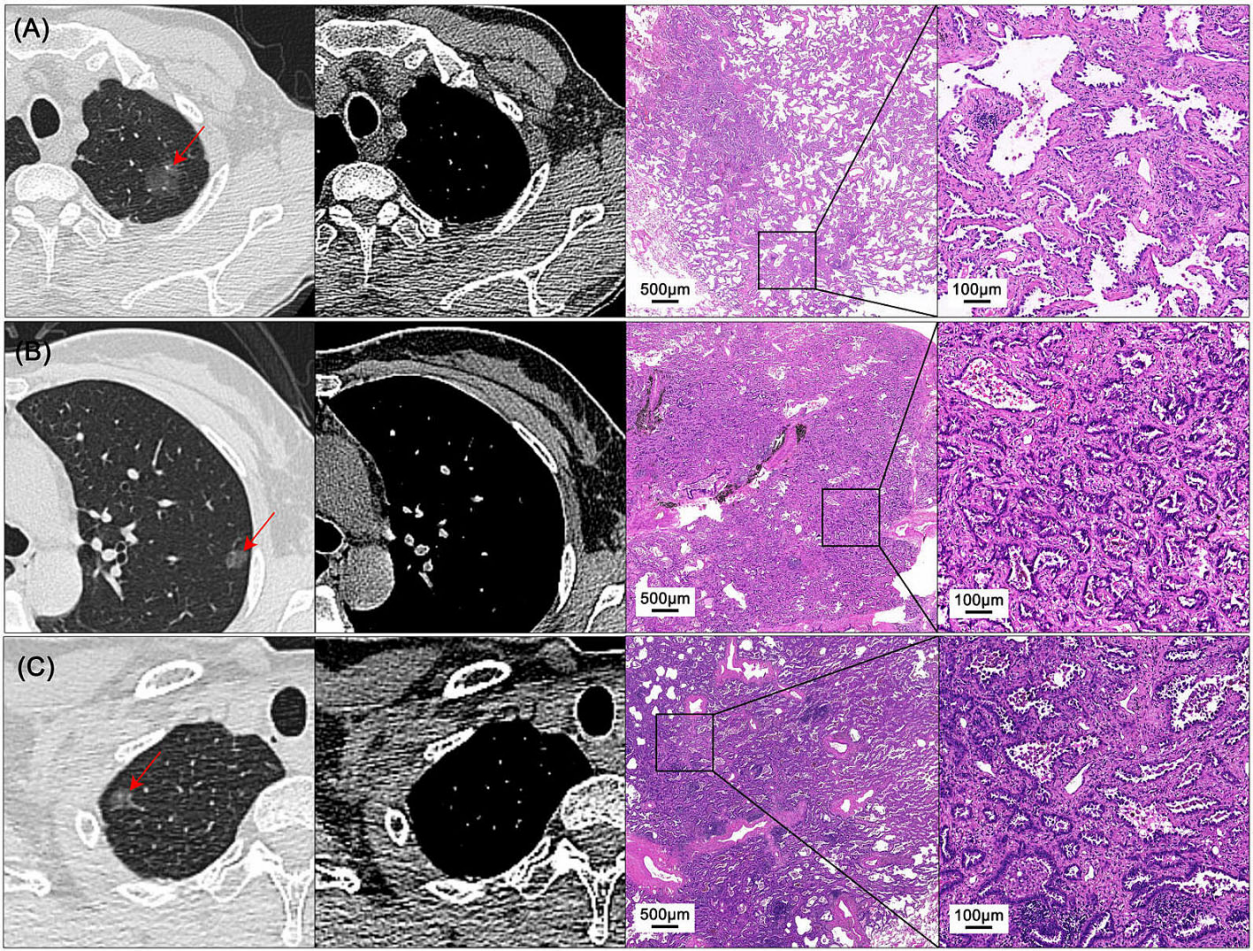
Example of the HRCT images and pathological images for pGGN‐featured IAC. (A) Axial HRCT images and pathological images show a pGGN in the left upper lobe of a 58‐year‐old man. (B) Axial HRCT images for a pGGN in the left lower lobe of a 40‐year‐old woman. (C) Axial HRCT images for a pGGN in the right upper lobe of a 59‐year‐old woman. There are no detectable solid components in all the above HRCT images. HRCT, high‐resolution CT; pGGNs, pure ground glass nodule.
